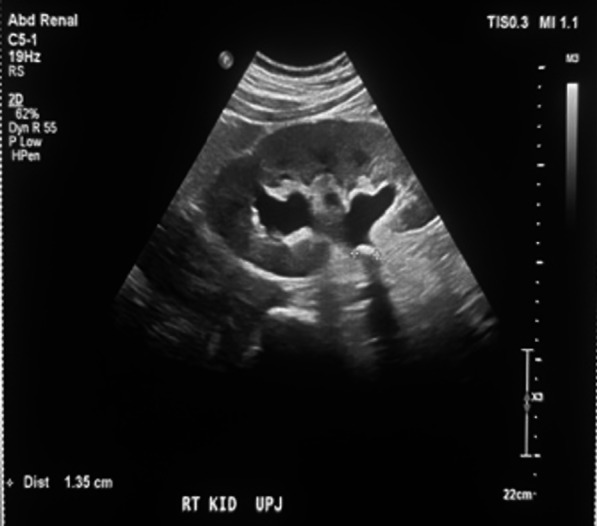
Renal ultrasound showing an obstructive 1.4-cm calculus at the right UPJ, causing moderate hydronephrosis
